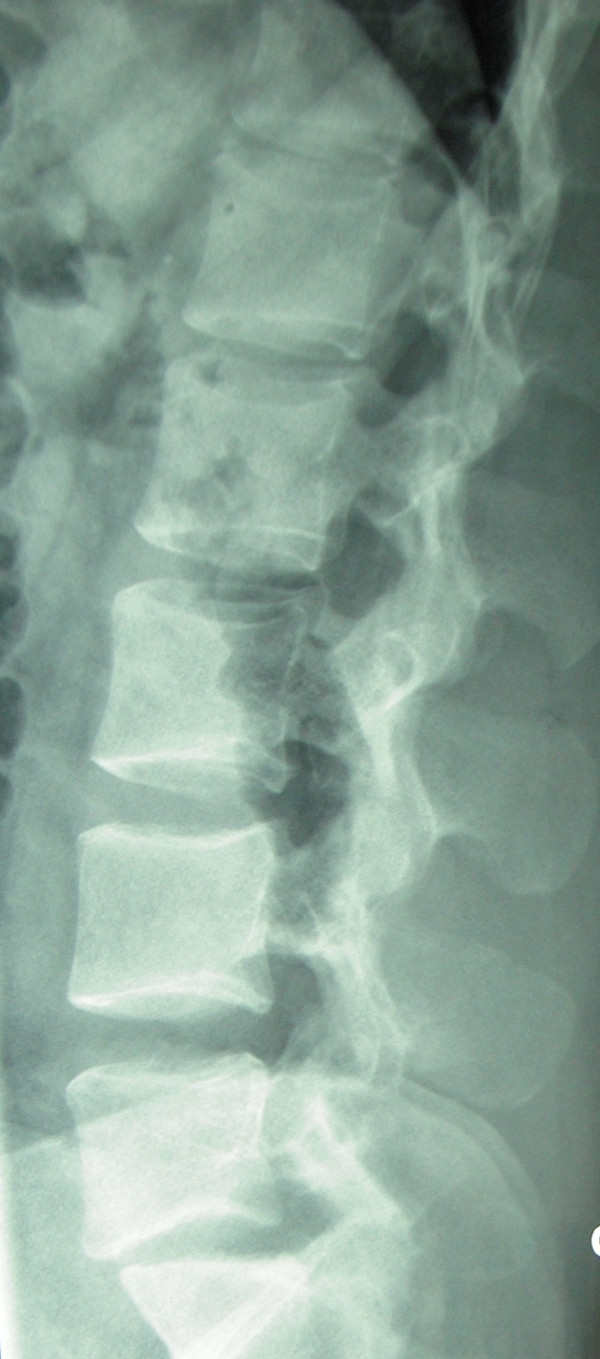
Lateral radiograph of the same patient.
